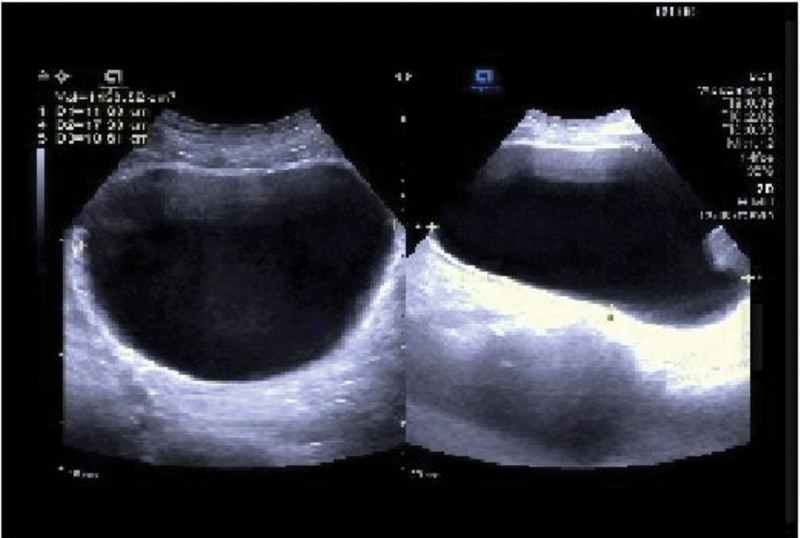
Urologic Doppler ultrasound showed: normal prostate size (3.9 cm*2.9 cm*2.6 cm); post voiding bladder residual urine: 1153 mL.
